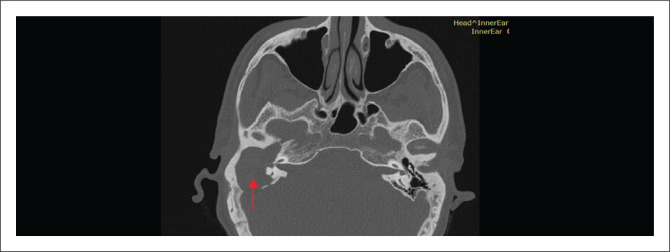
Right ear cholesteatoma in a 29-year-old male patient. Axial CT image demonstrating extensive bone erosions involving the labyrinthine capsule, ossicular chain and mastoid antrum walls (red arrow). Findings are consistent with advanced cholesteatoma.
